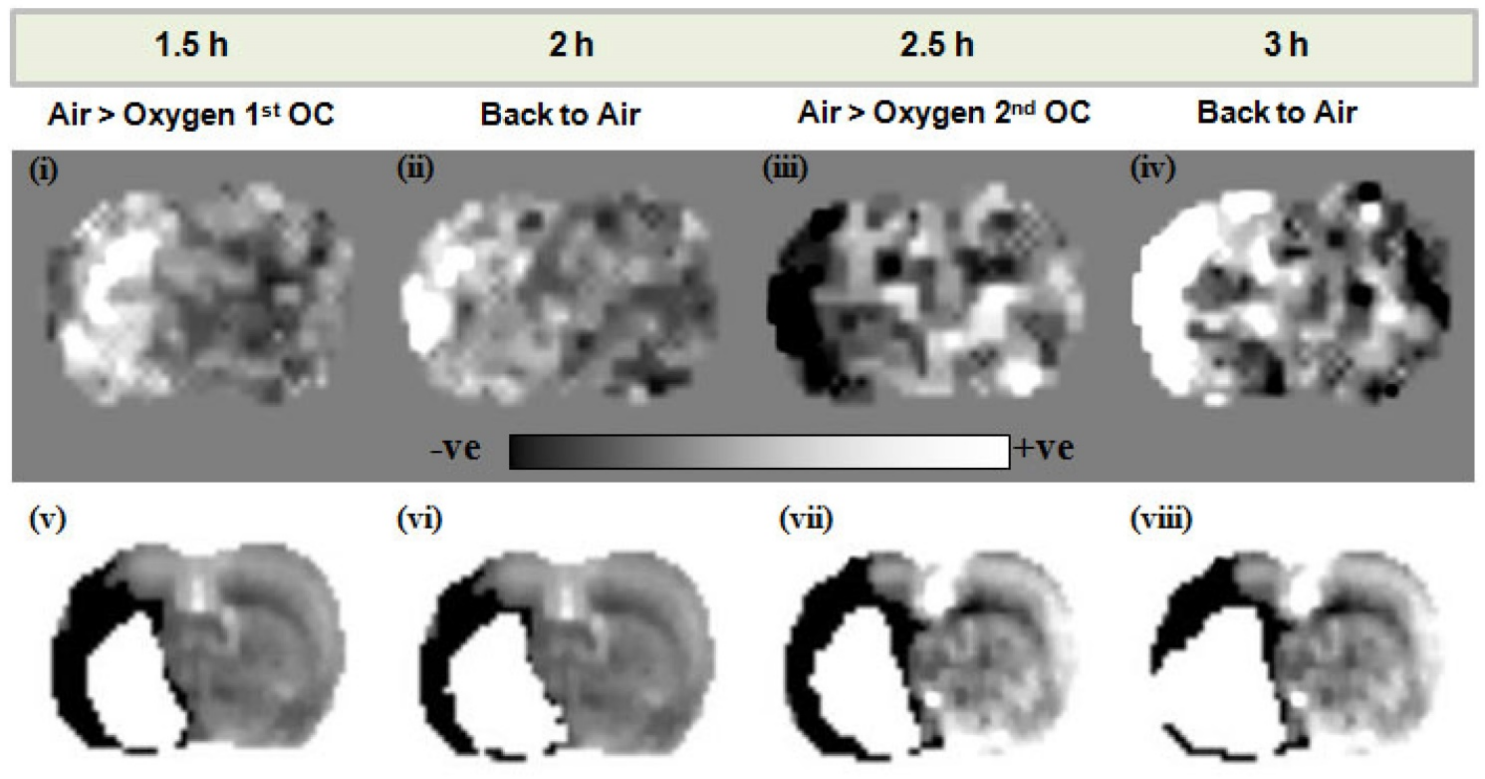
Lactate change aerobic (i, iii) and anaerobic (ii, iv) maps following administration of O-PFC (4.5 mL/kg) with time matched DWI/PI mismatch images (v-viii) for the first (1.5 h post-MCAO) and second (2.5 h post-MCAO) OCs. Regions of increased (white regions) or decreased (black regions) lactate evident in images i-iv were similar to but not exactly matching the region of DWI-PI mismatch shown in black in images v-viii. The white region in images v-viii represents the thresholded ADC lesion representing the ischaemic core. ADC: apparent diffusion coefficient; DWI: diffusion-weighted imaging; MCAO: middle cerebral artery occlusion; OC: oxygen challenge; PI: perfusion imaging.
